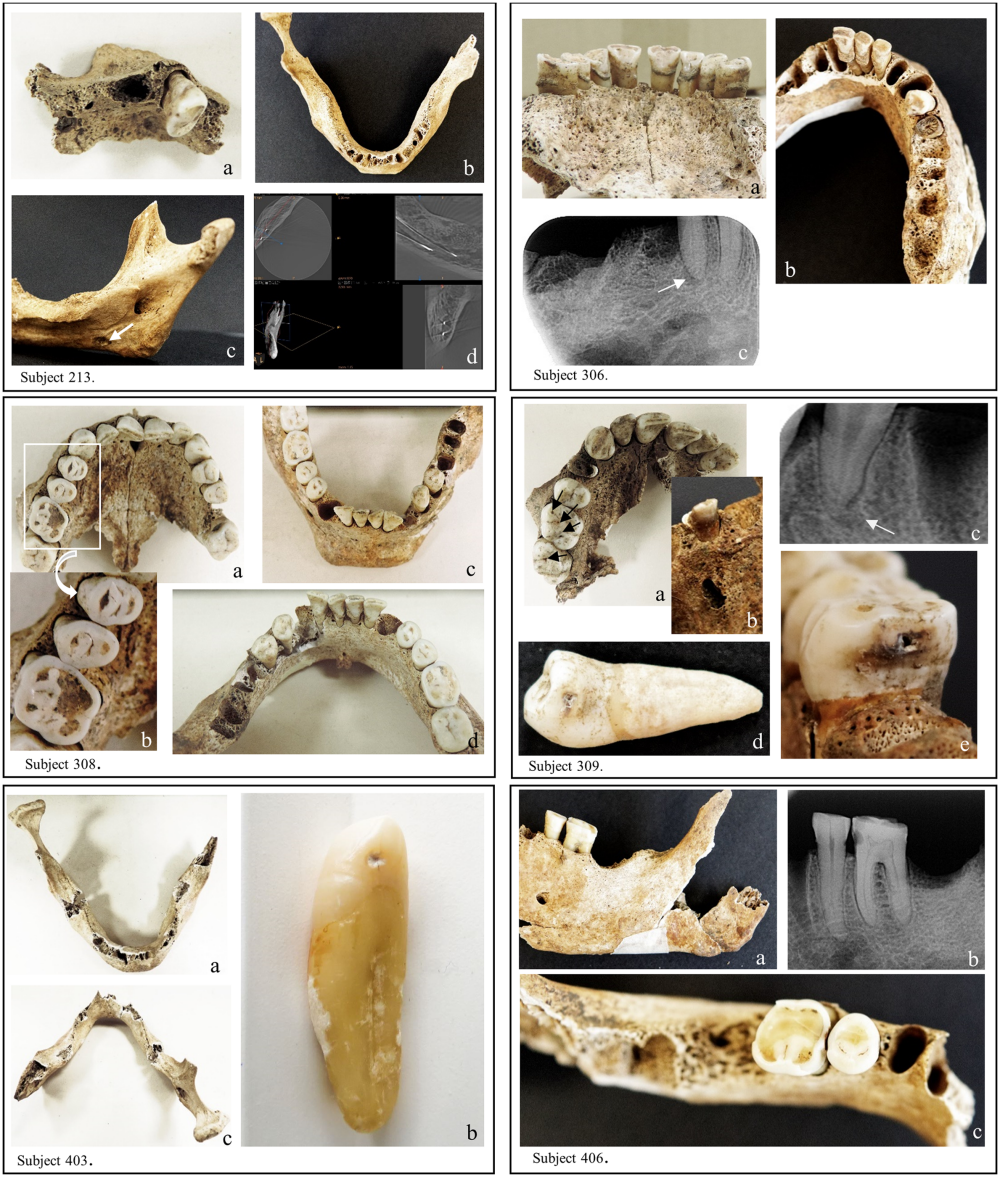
Photographs, X-ray and Cone Beam Computed Tomography (CBCT) of maxilla and mandible from sampled subjects. (Subject 213) a: maxillary septal region showing sharp, ragged aspect on both sides of tooth 14; b: deformation of the mandibular cortical bone on the right part of the horizontal branch; c: inner part of the mandibular right horizontal branch showing a fistula aperture (indicated by a white arrow); d: CBCT examination differentiating the fistula’s pathway and the inferior alveolar nerve pathway. (Subject 306) a: maxillary teeth presenting significant deposits of dental calculus; b: disorganized and riddled posterior mandibular septal morphology and destruction of the crown part of tooth 45; c: X-ray view of a granuloma on the apical part of tooth 45 (indicated by a white arrow). (Subject 308) a; c and d: sound teeth, maxillary and mandibular bones (dental calculus presents on lingual tables of teeth 31 ad 41; b: focus on important dental wear on teeth 14; 15 and 16. (Subject 309) a: groove decay on the occlusal tables of maxillary teeth 16 and 17 (indicated by black arrows); b: vestibular fenestration of the maxilla in front of the root apex of tooth 14; c: radiologically visible periapical cyst on the apex of tooth 14 (indicated by a white arrow); d: decay on the distal table of tooth 34; e: decay on the mesial table of tooth 46. (Subject 403) a and c: no teeth on the mandibular arch (except tooth 43 which was sampled for analysis); b: closer view of tooth 43 showing a small patch of distal decay. (Subject 406) a: external view of the left horizontal mandibular branch supporting teeth 35 and 36; b: retroalveolar X-ray image highlighting decay on distal table of tooth 35 and mesial table of tooth 36; c: occlusal view of teeth 35 and 36 revealing dental coloration due to the decay process between the two teeth. Pictures realized and assembled by C. Willmann.
